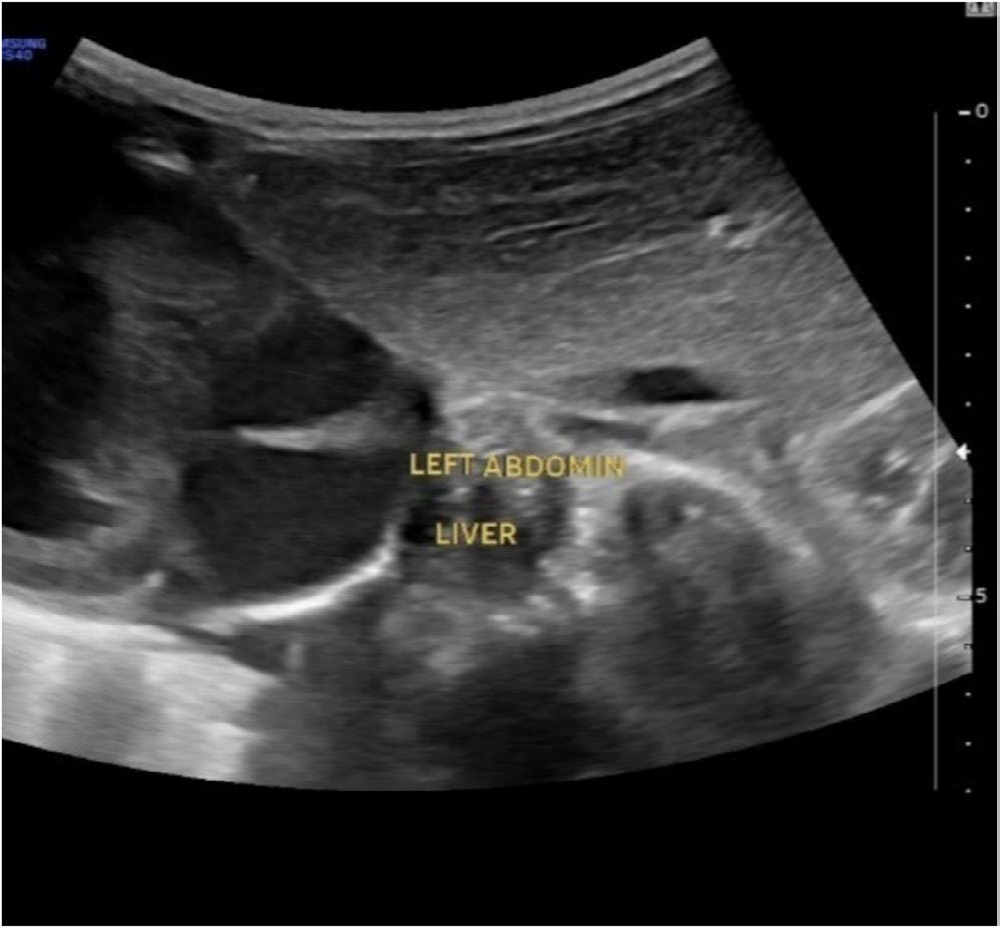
An abdominal ultrasound of the left upper quadrant reveals the liver on the left side, as opposed to its typical anatomical location.
